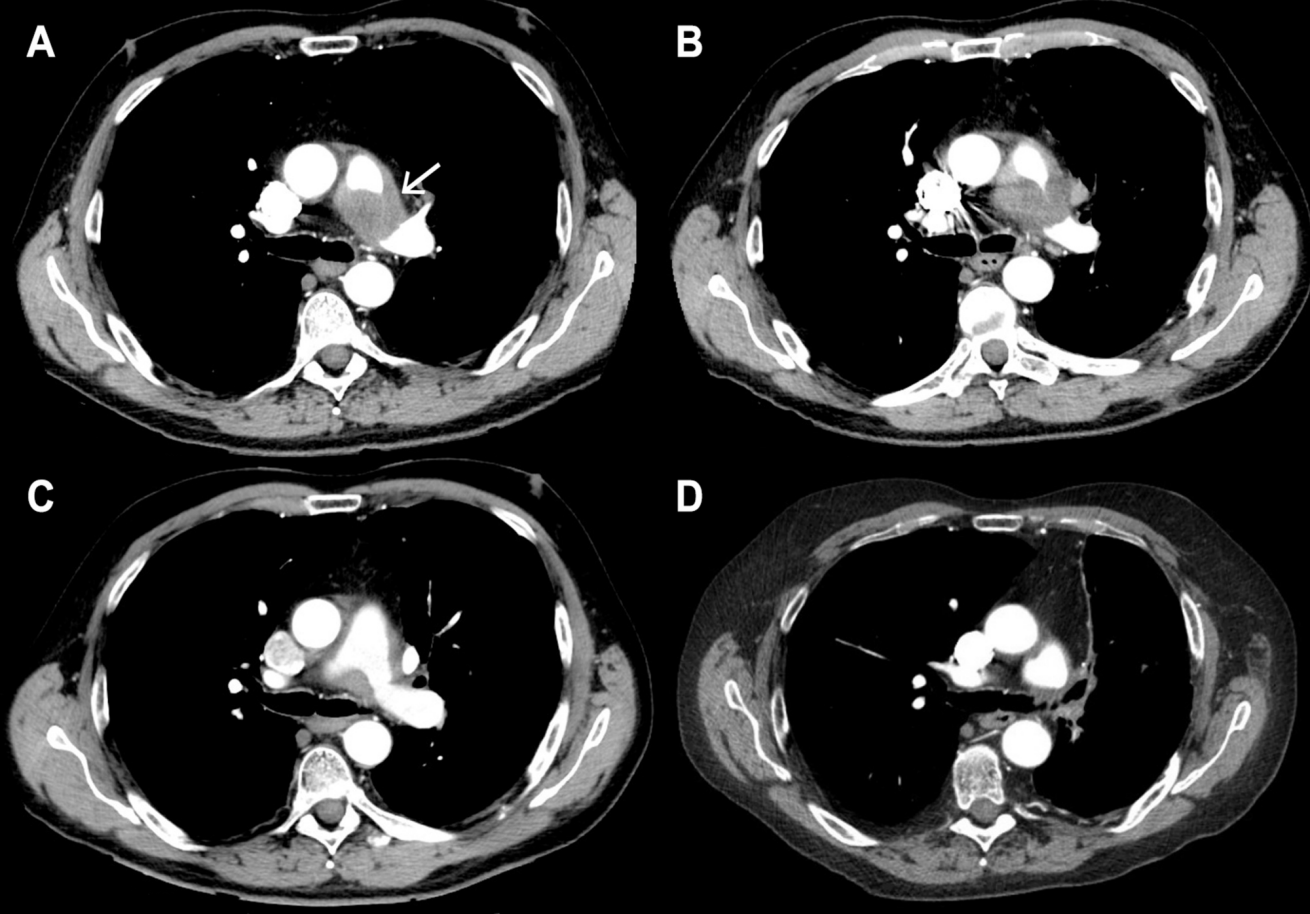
CT pulmonary angiogram images of left hilar mass causing pulmonary artery stenosis. (A) CT pulmonary angiogram from March 2012 revealed a new left hilar mass causing stenosis of the left pulmonary artery, measuring 4.5 x 3.5 cm. (B) By June 2012, the tumor had further enlarged to 5.1 x 3.9 cm. (C) One-month post-radiotherapy, the lesion had significantly reduced in size, and now measured 3.1 x 2.9 cm. (D) 7.3 years post-radiotherapy, the residual fibrotic mass remains minimal.
